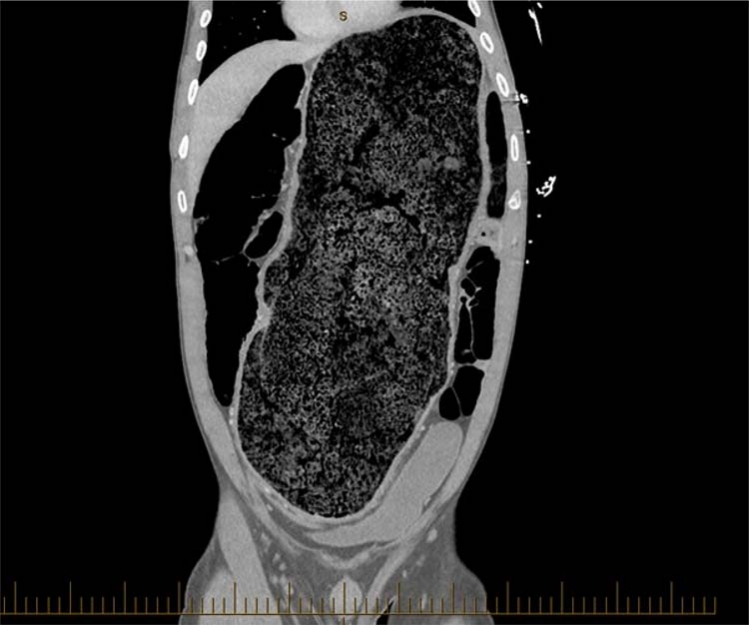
Coronal view of a CT image of a large faecaloma in the sigmoid.
